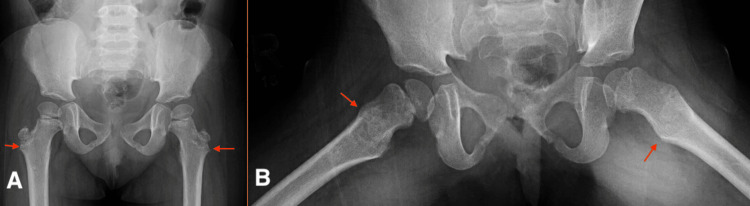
AP pelvis (A) and frog legs lateral (B) X-ray. Bilateral iliac crest as well as femoral metaphyseal irregularity (red arrows), likely related to the known renal disease. Visualized bones and joint spaces are unremarkable. No fracture or dislocation. No gross soft tissue abnormality. AP, anteroposterior
